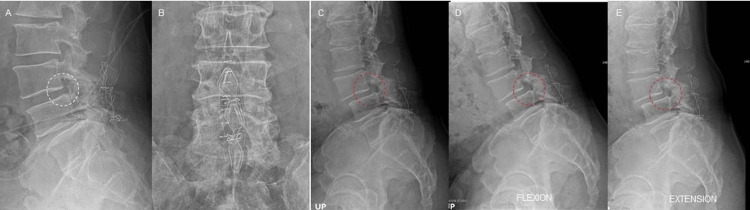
Case 2 Progression of Slippage Postoperative ILP X-ray lateral (A) and AP (B) lumbar X-ray views taken two months after the first surgery, showing the artificial ligament placed around spinous processes with preserved segmental motion and minimal translation (A, white circle). Seven months postoperative dynamic views (C-E) showing progression of slippage (red circle). ILP: interspinous ligamentoplasty, AP: anteroposterior.
